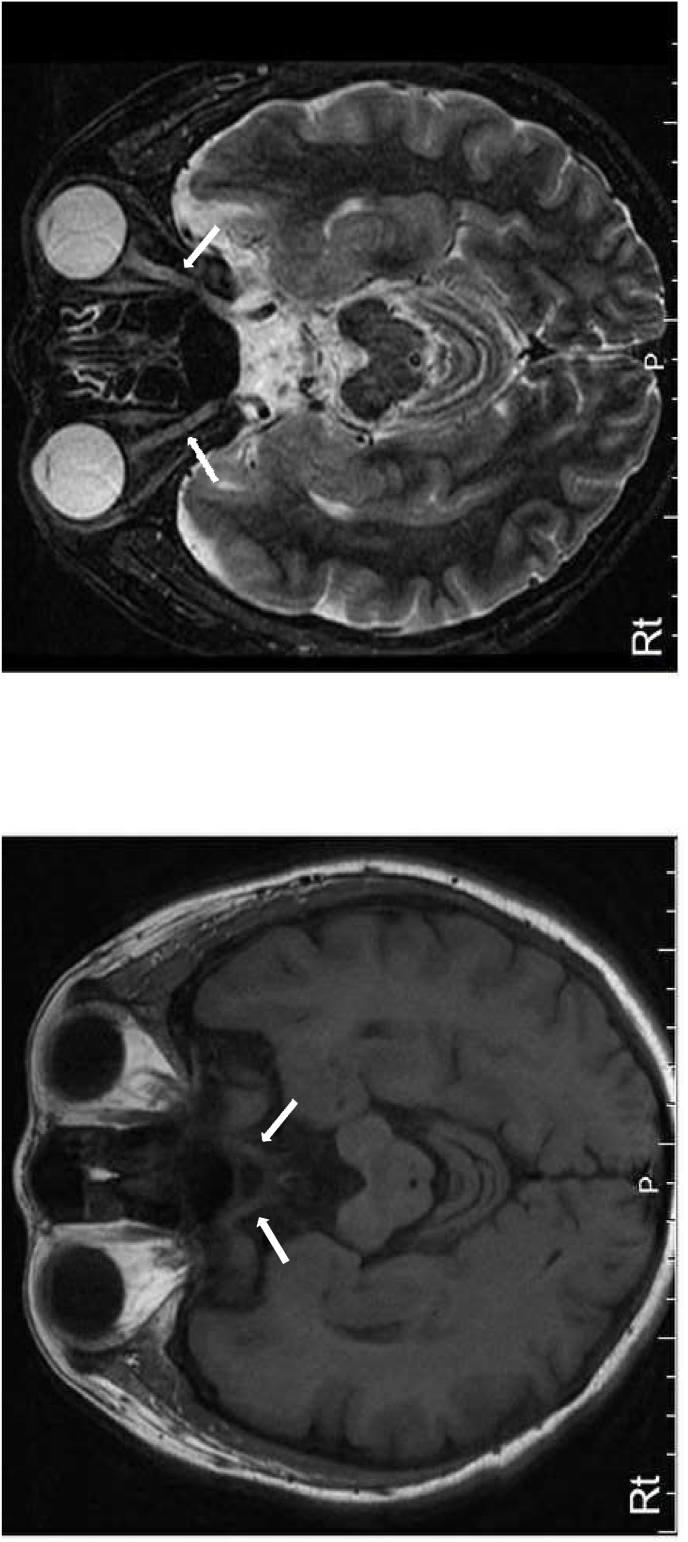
The magnetic resonance imaging of the optic tract and optic chiasm. Magnetic resonance imaging demonstrates bilateral optic atrophy from the optic nerves (arrows) to the optic tract. Right panel: Fluid-attenuated inversion recovery (FLAIR); Left panel: short TI inversion recovery (STIR). Rt: Right.
